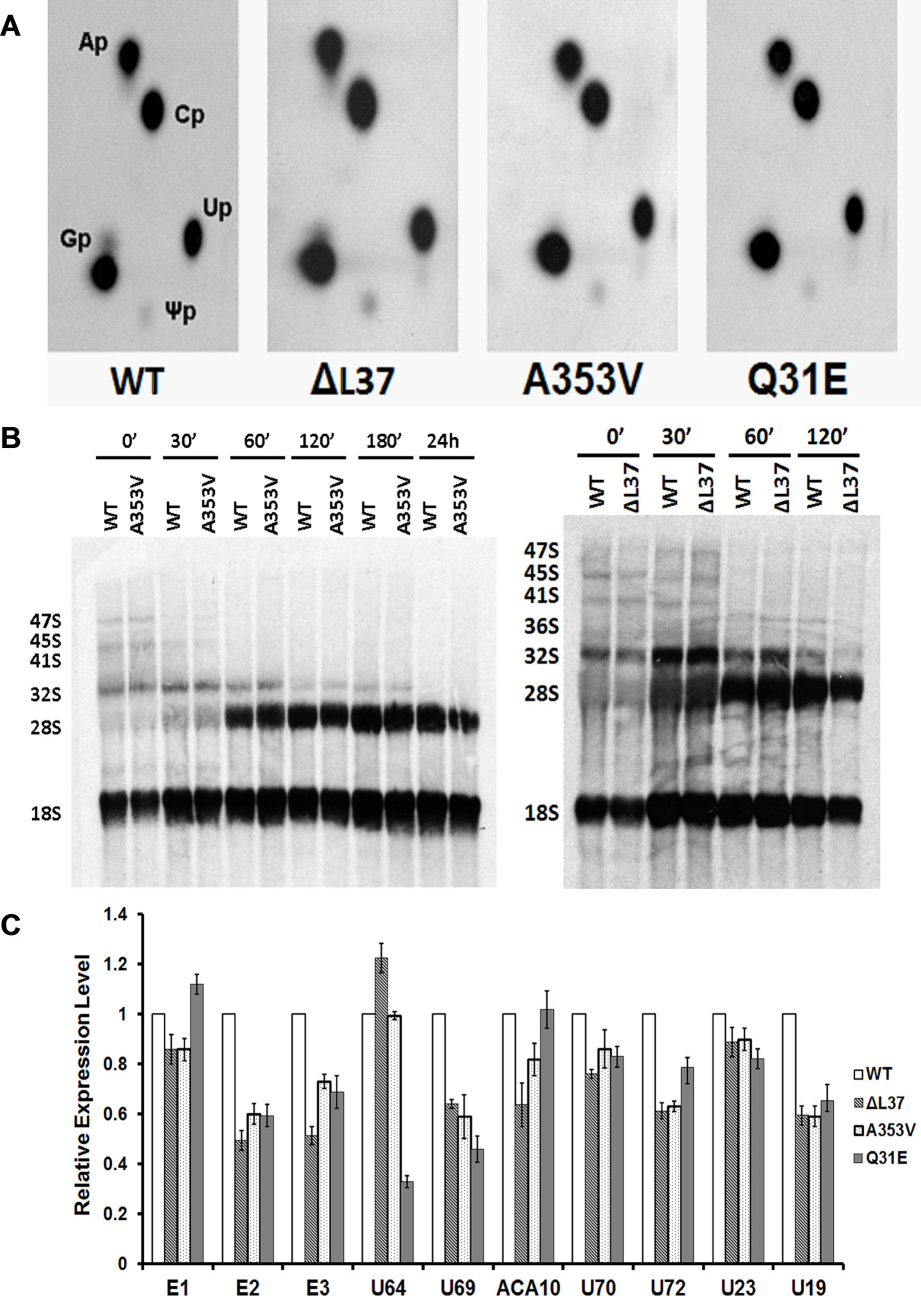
DKC1* mutant iPS cells show no significant defects in ribosome biogenesis. A: measurement of pseudouridine in 28S rRNA from WT and mutant iPS cells. iPS cells were labeled with ^32^P-labeled orthophosphate and 28S rRNA was gel-purified. After digestion with RNase T_2_, each sample was separated by two-dimensional TLC. The positions of the labeled ribonucleotides are indicated. Ap: Adenine, Cp: Cytosine, Gp: Guanine, Up: Uridine, Ψp: Pseudouridine: B: Pulse–chase labeling experiments of rRNA isolated from *WT*, *A353V* and *ΔL37* iPS cells. Cells were labeled with L-[^3^H-methyl] methionine for 30 min and then chased in nonradioactive medium for the times shown. The RNA was separated on a 1.25% agarose gel, transferred to a nylon filter, and exposed to x-ray film. C: Real-time RT/PCR results of some H/ACA snoRNAs of *WT* and *DKC1* mutant iPS cells. Results were expressed relative to *GAPDH* RNA. The combined results of 3 independent experiments are shown, the error bars show standard deviation.
